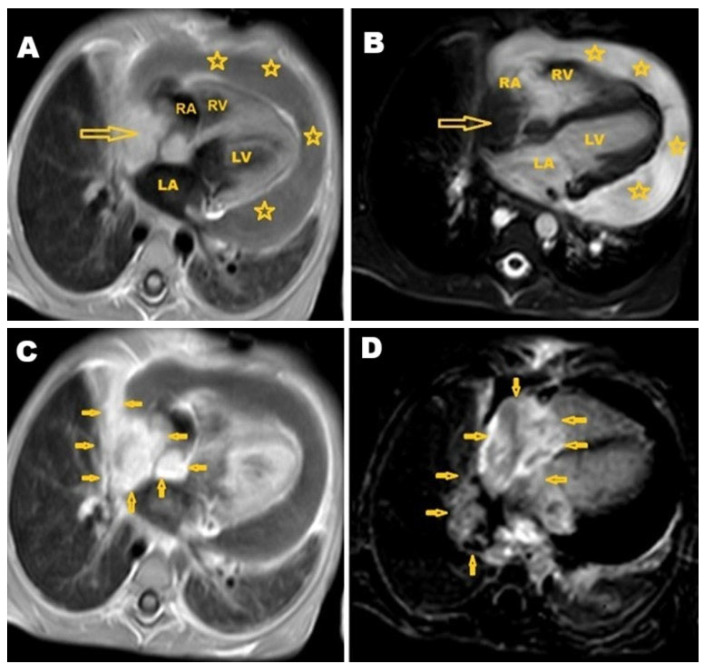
Selected cardiac MRI images. (A) Axial T1 4-chamber view shows isointense infiltrative lesion in the right heart wall (arrow) invading the right atrium (RA) with a large amount of low-signal-intensity pericardial effusion (stars); (B) T2 4-chamber view shows a hypointense mass lesion in the right wall of the heart (arrow) invading the RA, with a large amount of high-signal-intensity pericardial effusion (stars); (C) T1 post-contrast 4-chamber view shows large ill-defined mass lesion infiltrating the right side of the mediastinum, invading the right pericardium, right atrial wall, and interatrial septum, and associated with large pericardial effusion; and (D) late gadolinium enhancement (LGE) 4-chamber view shows enhancement of the lesion. MRI: magnetic resonance imaging, RA: right atrium, LA: left atrium, RV: right ventricle, LV: left ventricle.
